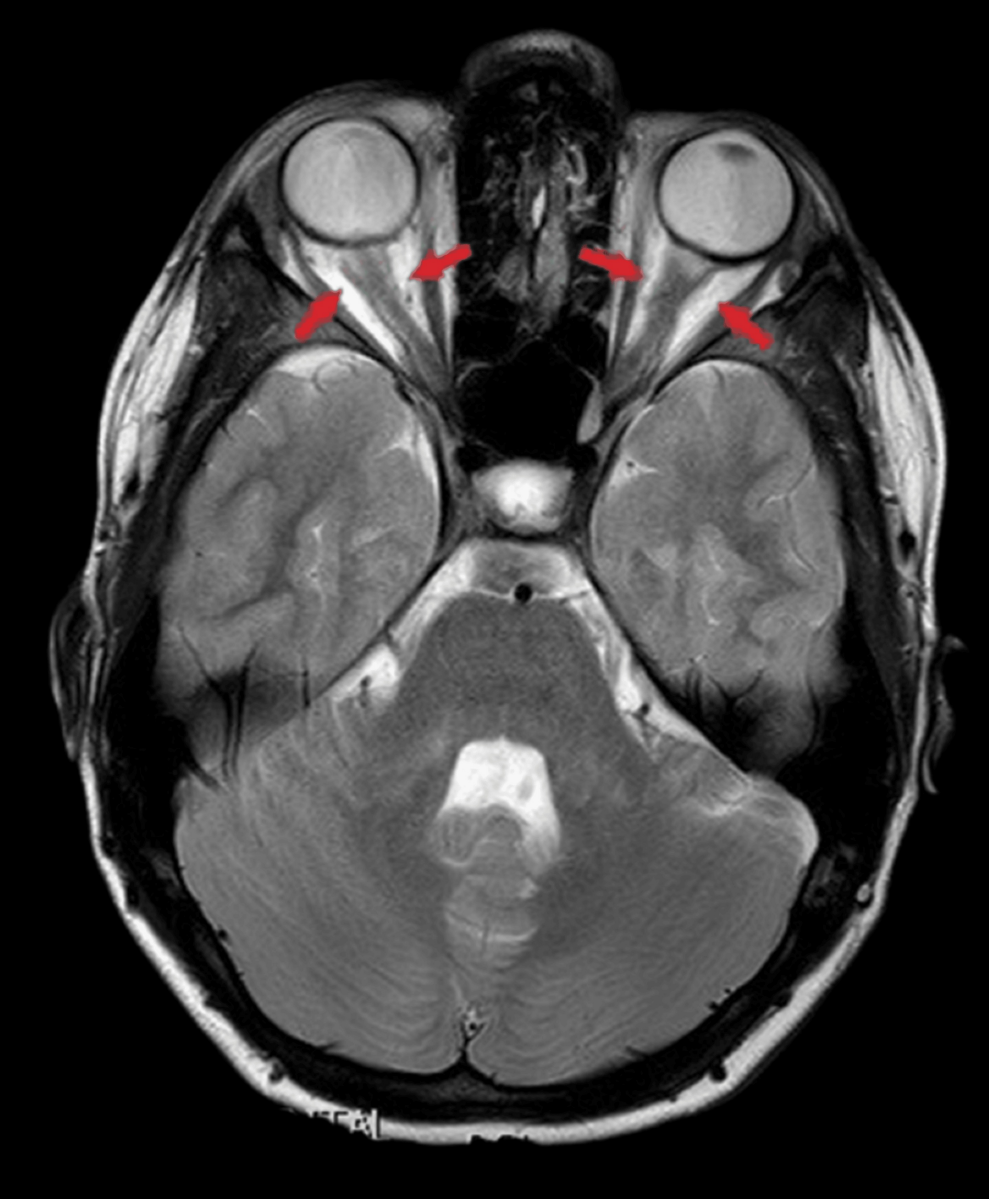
Axial contrast-enhanced magnetic resonance imaging (MRI) of the orbits showing bilateral circumferential enhancement of the optic nerve sheaths (Tram-Track sign, indicated by red arrows), consistent with optic perineuritis.
